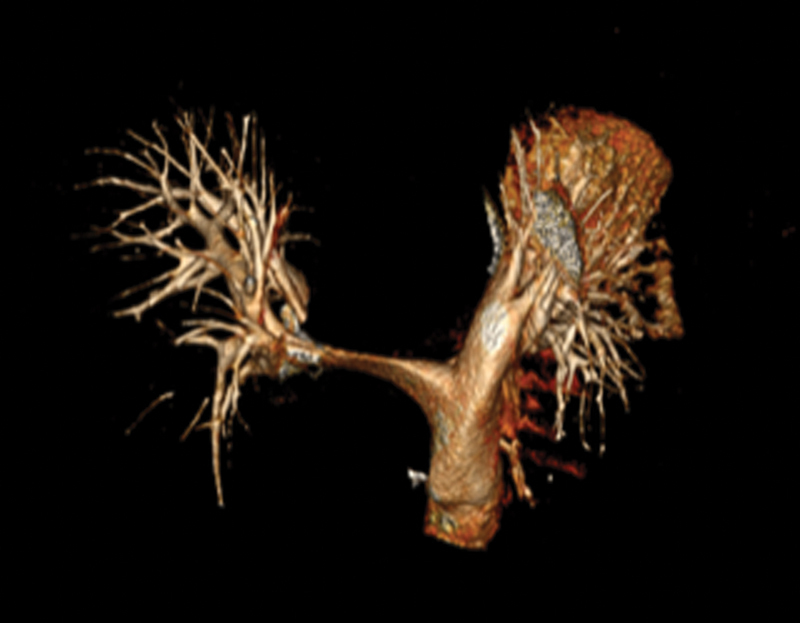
Three-dimensional computed tomography reconstruction of pulmonary artery system showing extreme narrowing of the right pulmonary artery.
